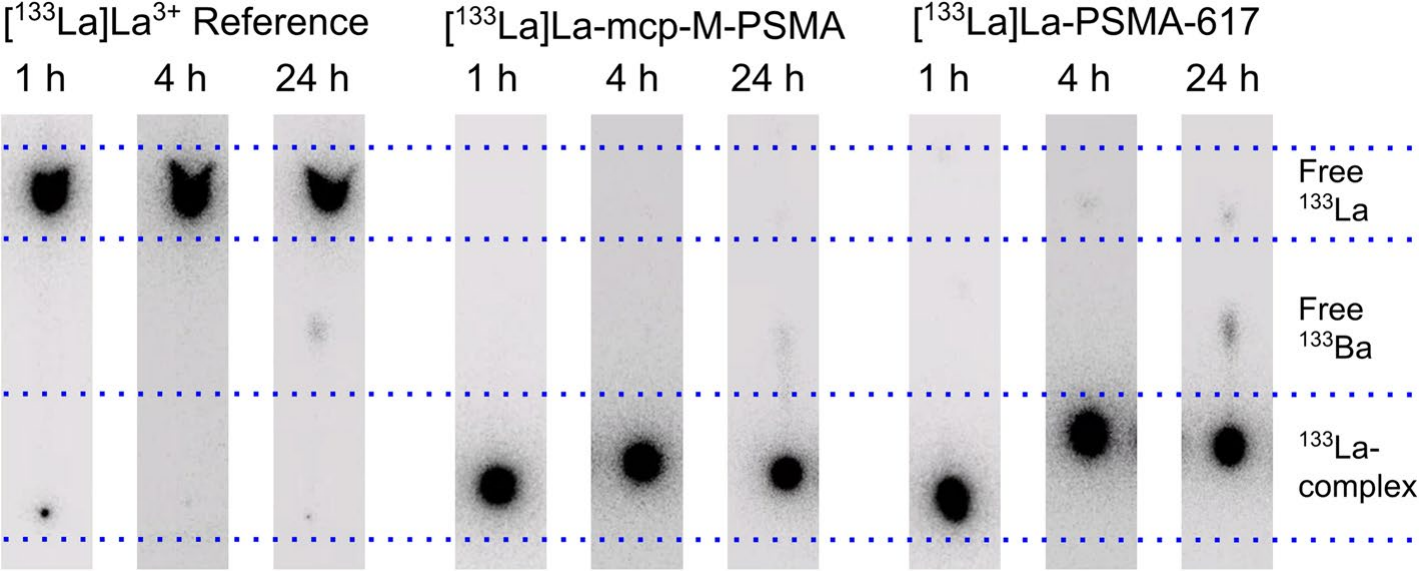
Human serum stability results for [^133^La]La-PSMA-617 and [^133^La]La-mcp-M-PSMA (80 GBq/µmol) after 1 h, 4 h and 24 h. Uncoordinated [^133^La]LaCl_3_ is included as reference
